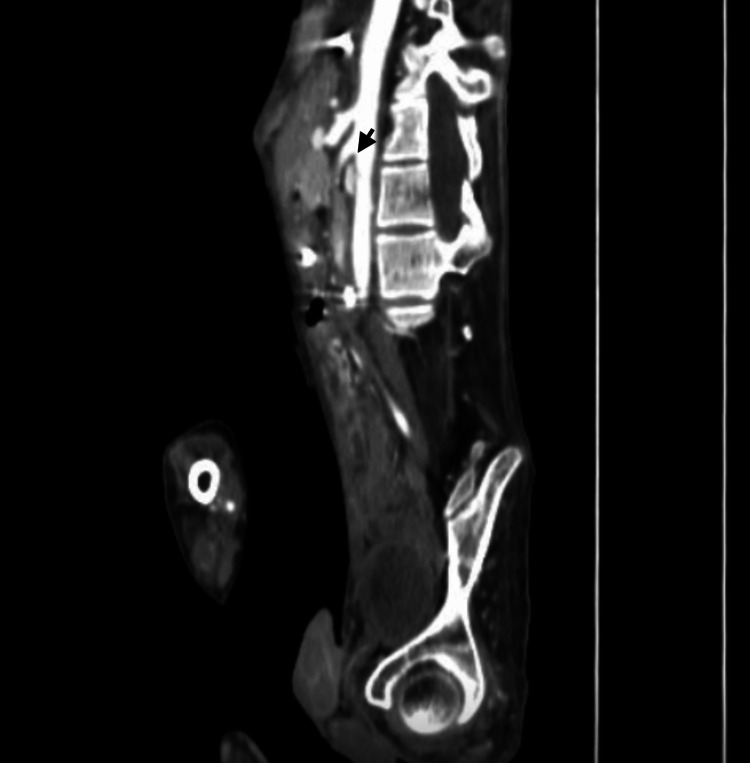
Abdominopelvic CT scan (sagittal view) showing reduced dilation of the stomach and duodenum, while the narrow aortomesenteric distance remains unchanged (black arrow) CT: computed tomography
